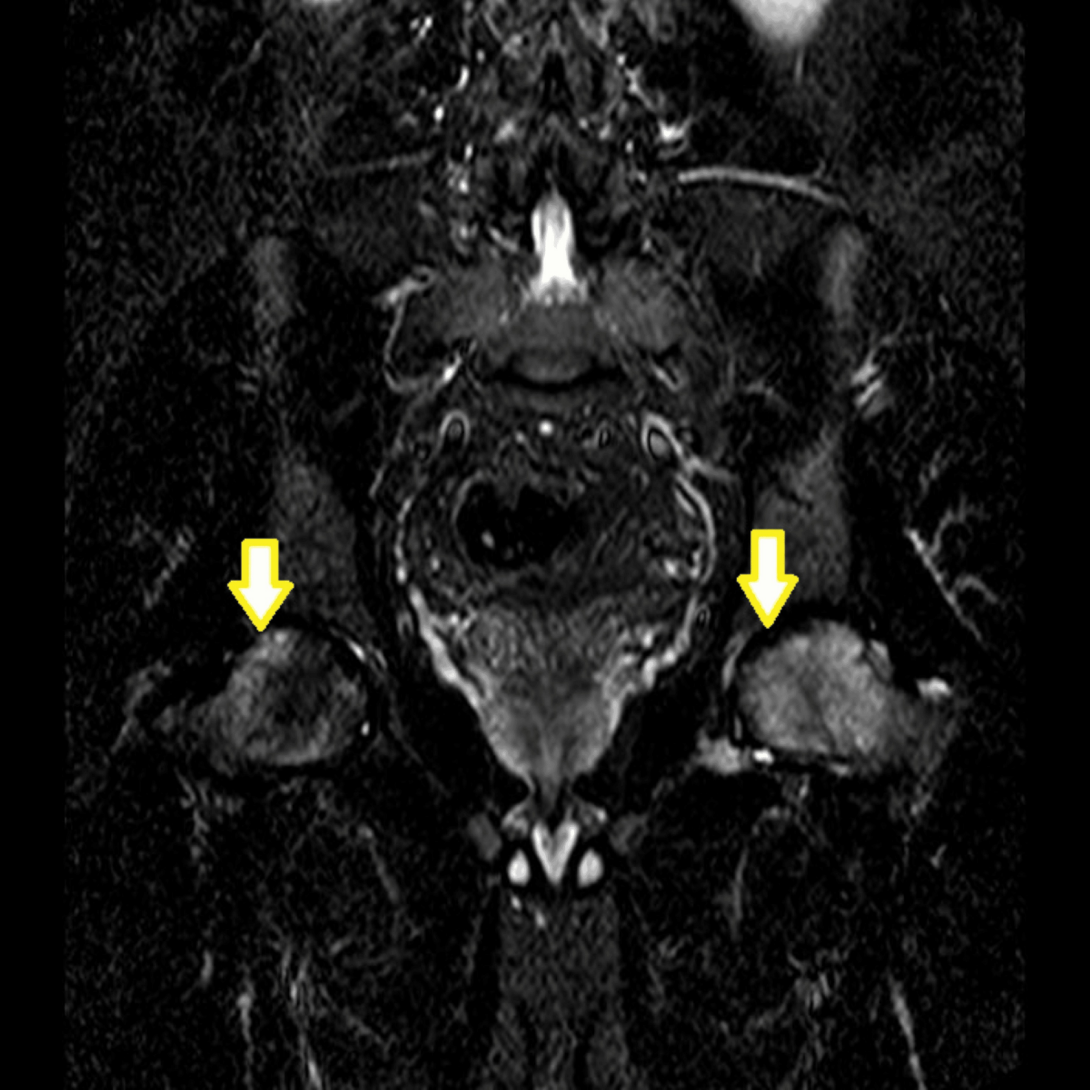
Coronal T2-weighted short tau inversion recovery (STIR) MRI of the pelvis. The image reveals extensive, high-signal intensity bone marrow edema involving both femoral heads. Note the presence of the pathognomonic "double-line sign"; a low-signal peripheral rim of sclerosis with an inner high-signal band of granulation tissue, demarcating the necrotic segment. These findings confirm bilateral avascular necrosis with early cystic changes and volume loss, more pronounced on the left side.
